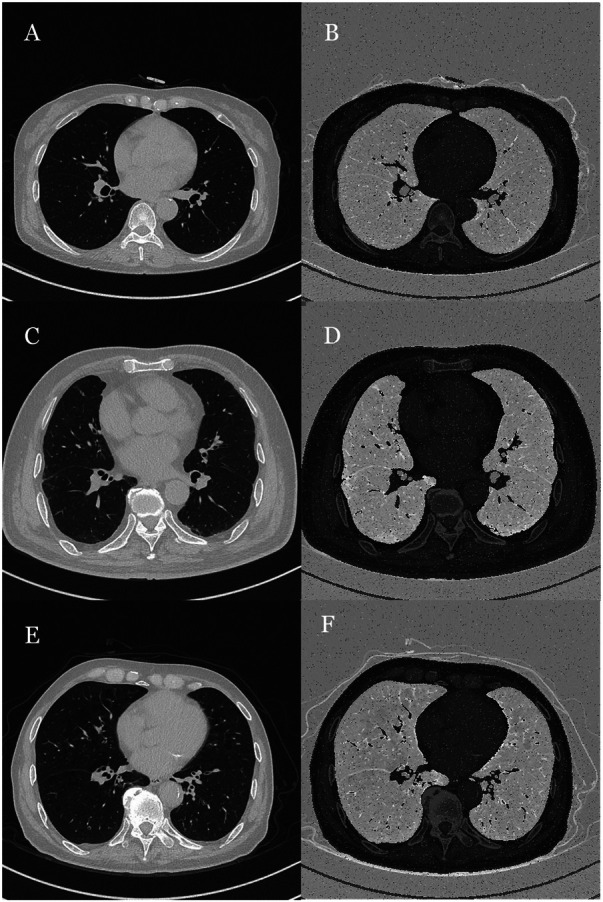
Original and preprocessed chest CT images. (A) Original axial chest CT image (lung window) of a normal lung. (B) Preprocessed normal chest CT slice after window adjustment (1,500/−450), wavelet denoising (db1), and convolutional denoising autoencoder enhancement. (C) Original axial chest CT image (lung window) of a PRISm case. (D) Preprocessed PRISm chest CT slice after identical preprocessing. (E) Original axial chest CT image (lung window) of a COPD case. (F) Preprocessed COPD CT slice after identical preprocessing. All images were standardized to 512 × 512 resolution. PRISm, preserved ratio impaired spirometry; COPD, chronic obstructive pulmonary disease.
